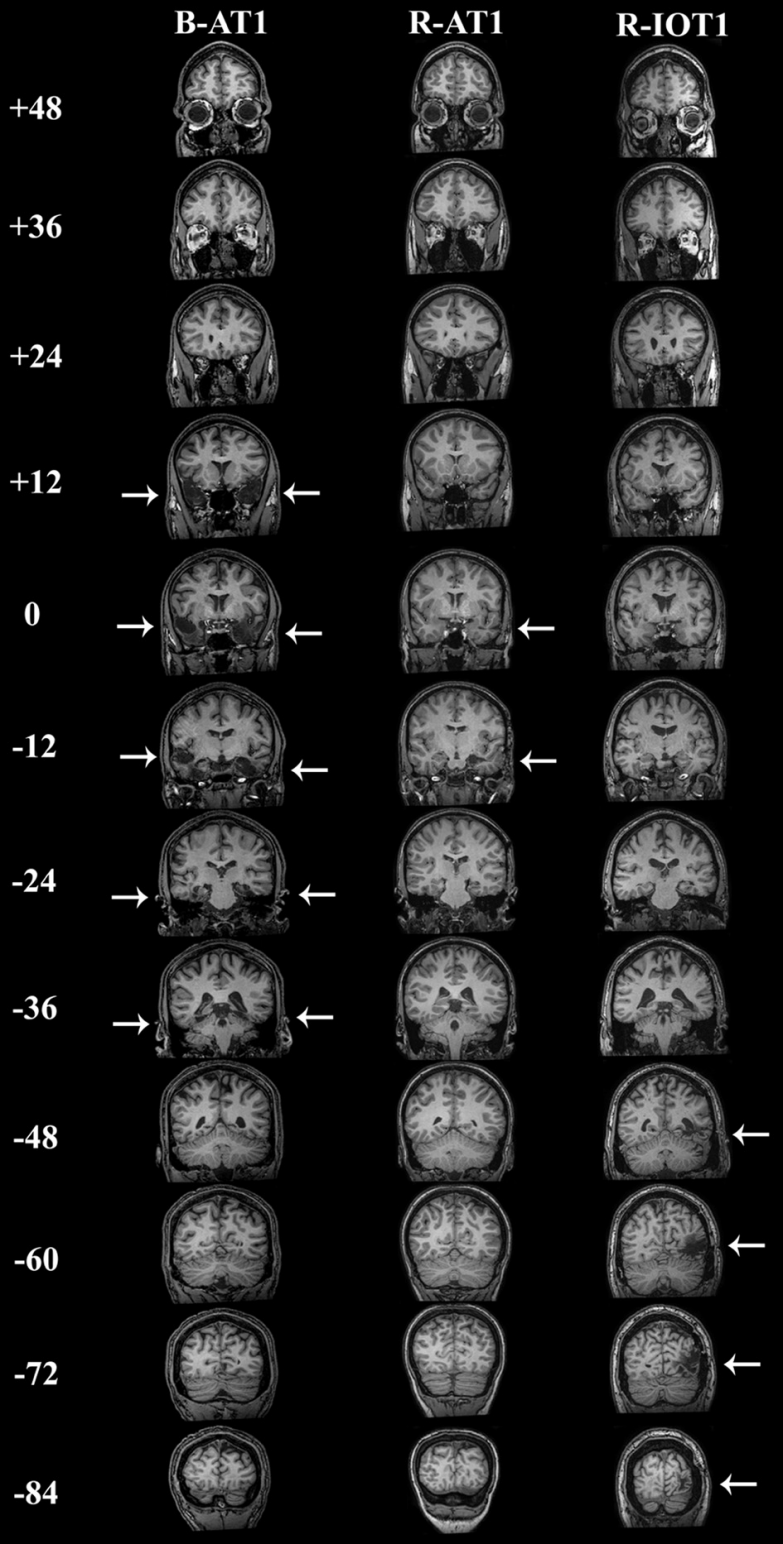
Coronal T1-weighted MRI brain images of the three patients, standardized to Talairach space. Slices were taken every 12 mm, from *y* = + 48 mm to *y* = −84 mm. B-AT1 has large bilateral lesions of the anterior temporal lobes following herpes encephalitis (+12 to −36 mm). R-AT1 has a small surgical lesion in the right anterior temporal lobe, additionally affecting the right hippocampus and amygdala (0–12 mm). R-IOT1 has a single right inferior occipitotemporal lesion from his prior hemorrhage (−48 to −84 m).
